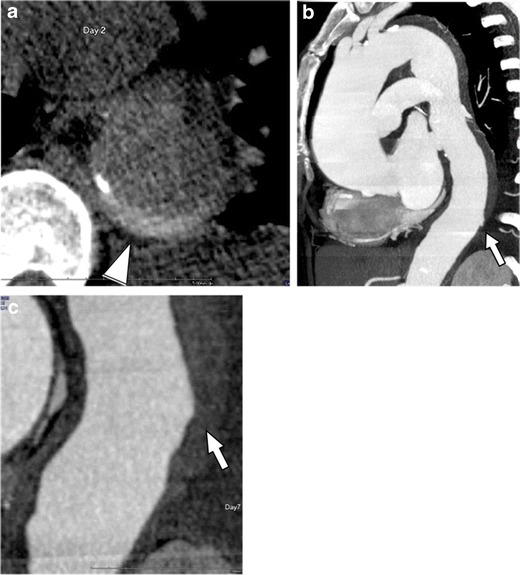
Resolved tiny primary intimal tear of dissection variant IMH in a 68-year-old woman with hypertension who presented with unstable thoracoabdominal pain. a Non-contrast CT image shows a crescent-shaped high-density area in the descending aorta (*arrowhead*). b Slab MIP image demonstrates haematoma extending through the whole thoracic descending aorta, which consists of dissection variant IMH. A tiny PIT is detected at the distal end of the haematoma (*arrow*). c The patient was followed with stable limited chest pain. After 7-day follow-up, the PIT resolved spontaneously, leaving a slightly enlarged haematoma (*arrow*). The patient was treated conservatively, and the haematoma eventually resolved
